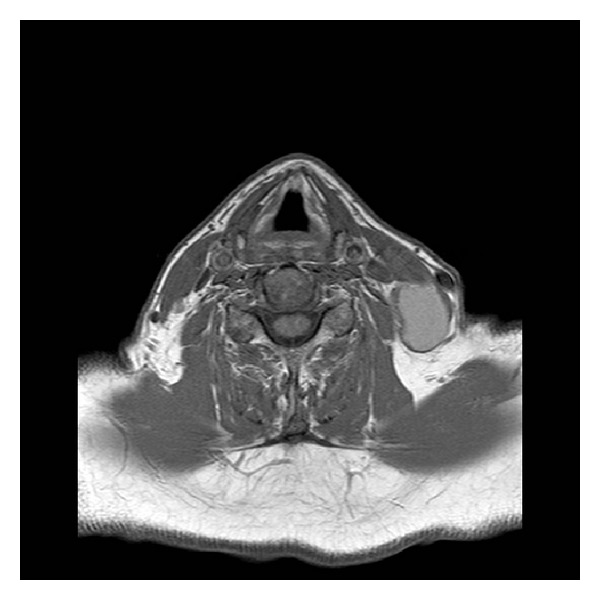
Axial slides of T1-weighted MRI showing a hyperintense cystic lesion behind the left sternocleidomastoid muscle in the neck level five.
